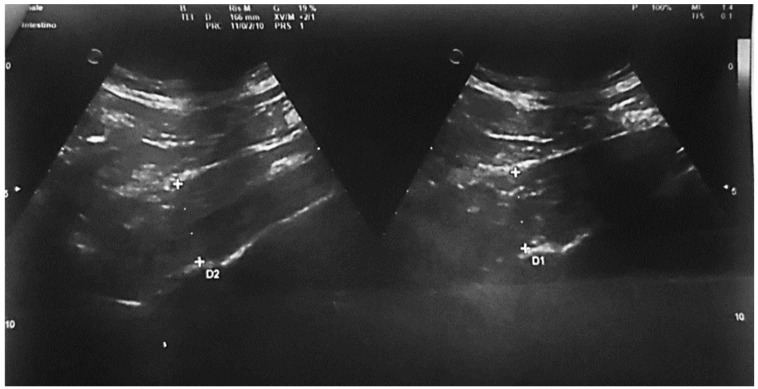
Muscle US measurement. Right psoas muscle and concomitantly lying lumbar vertebrae were accurately recognized in each patient. Abdominal probe was positioned in a sagittal plane subphrenally right and just above the upper anatomical limit of the pelvis (iliac crest). Sliding the ultra-sonic probe in the anteromedial to posterolateral direction, the largest ultrasonically visible psoas muscle diameter was defined.
